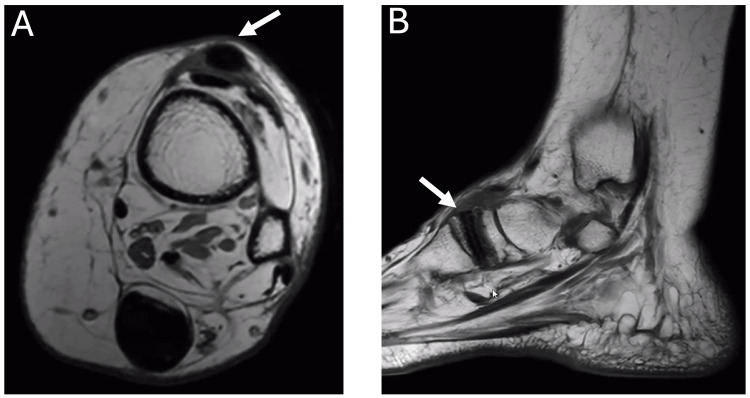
MRI of left ankle, October 2025 (A) Axial view showing the subcutaneous transfer of the PTT on the anterior ankle. (B) Sagittal view demonstrating integration of the tendon-bone interface postoperatively. White arrows indicate the PTT transfer in the left foot. PTT, posterior tibial tendon
